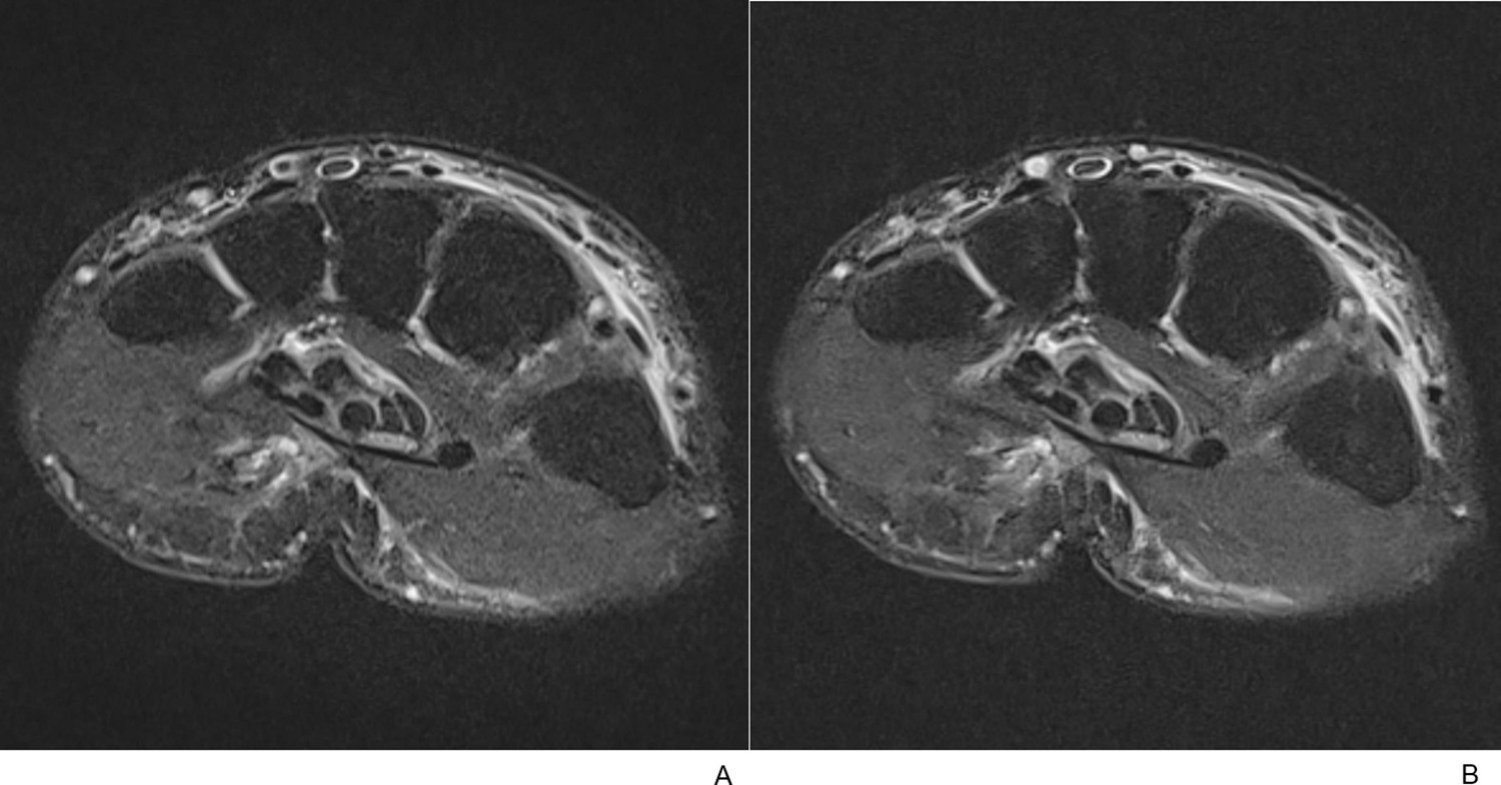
A 57-year-old female with a distal radius fracture. (A) Axial T2-weighted TSE_S_ image with fat suppression and (B) the corresponding TSE_DL_ image. TSE_DL_ images showed superior rSNR, rCNR and rCR compared to TSE_S_ images.
